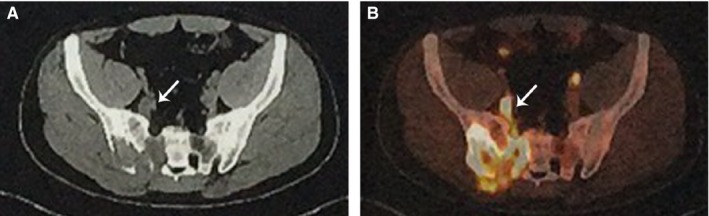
Manifestations of venous tumor thrombus (VTT) on PET/CT scan. A, Plain CT scan showed an osteosarcoma located at the right sacroiliac joint with a slightly enlarged internal iliac vein with low dense fillings (arrow), indicating a VTT within the internal iliac vein. B, PET scan showed high metabolic activity at the right sacroiliac joint and at the site of right internal iliac vein (arrow)
